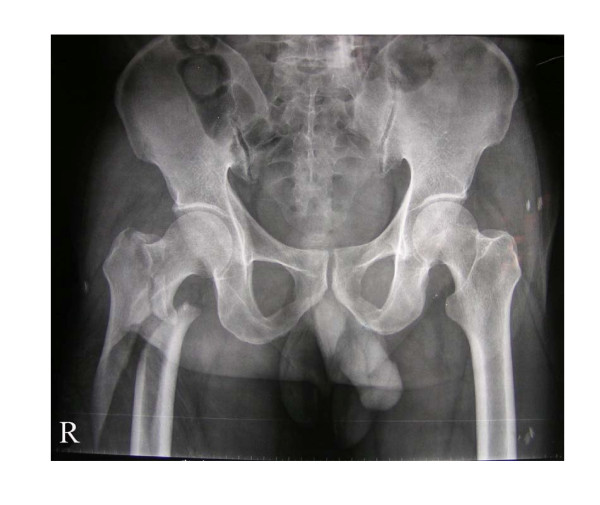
Initial radiograph of one representative case showing unstable intertrochanteric and subtrochanteric fractures of the right femur.
